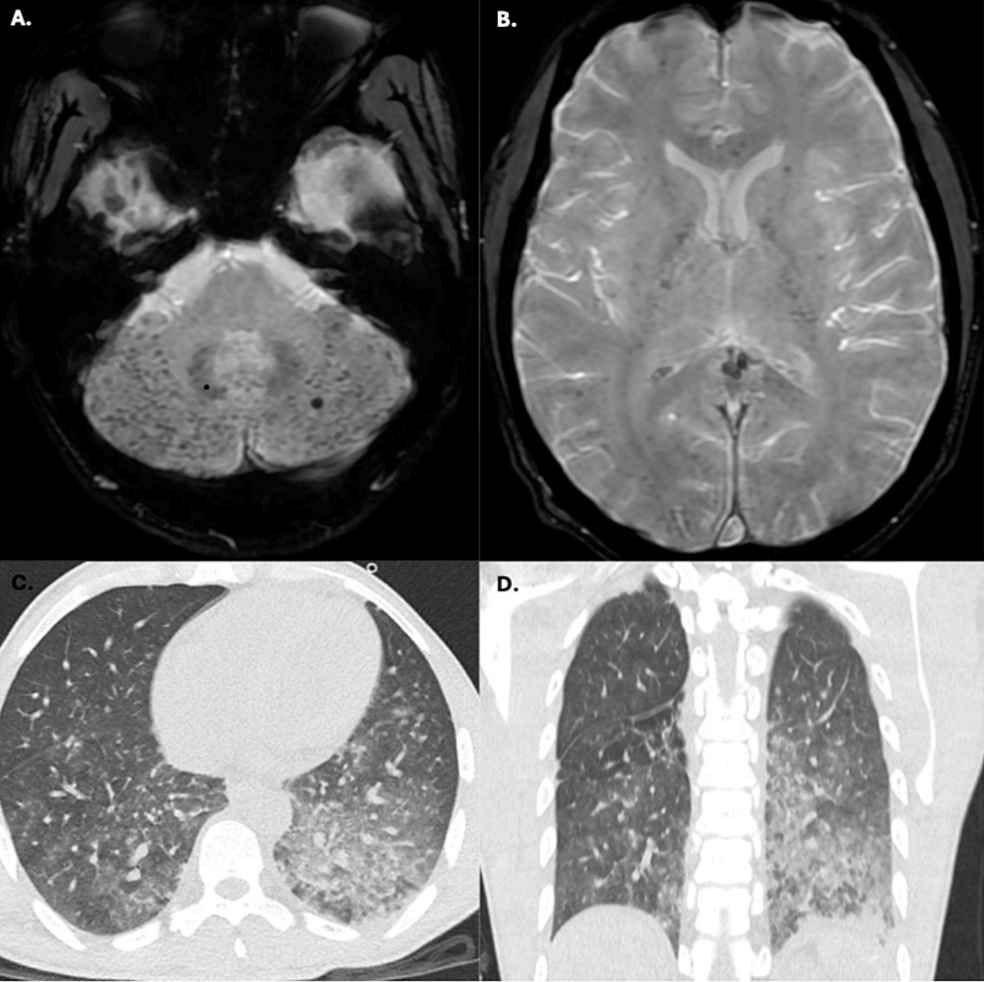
MRI and plain chest CT A-B: Countless microhemorrhages, predominantly subcortical, diffusely involving the cerebellum and, to a lesser extent, the cerebral parenchyma, the corpus callosum, and the corticospinal tracts. C-D: Alveolar opacities at the posterior basal segment of the left lung. Ground-glass opacities in both lower lobes. Numerous nodules and micronodules with a centrilobular distribution. MRI: magnetic resonance imaging, CT: computed tomography
